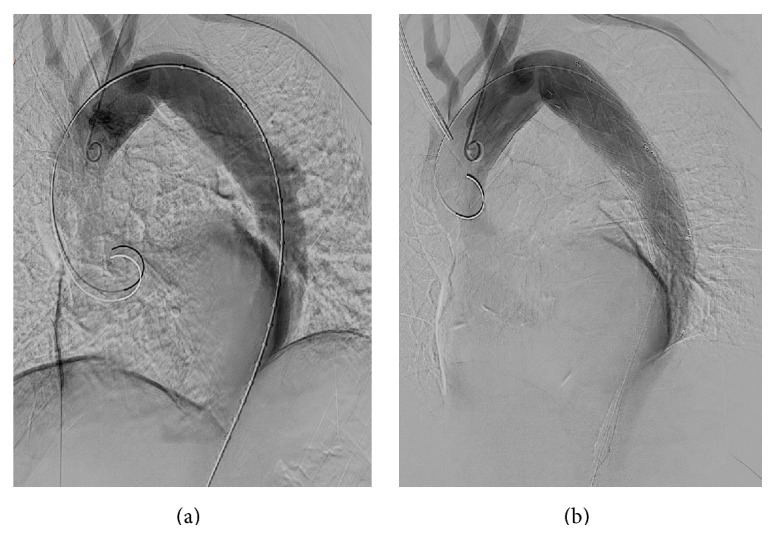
Image of angiographic findings taken during operative treatment. (a) Angiographic findings before treatment. The origin of the right subclavian artery was confirmed, and ULP was observed on contrast-enhanced CT. (b) Angiographic contrast-enhanced CT findings after placement of the stent grafts. The stent grafts were inserted so that the tips did not come into contact with the curve of the arch. The disappearance of the ULP was confirmed by contrast-enhanced CT.
